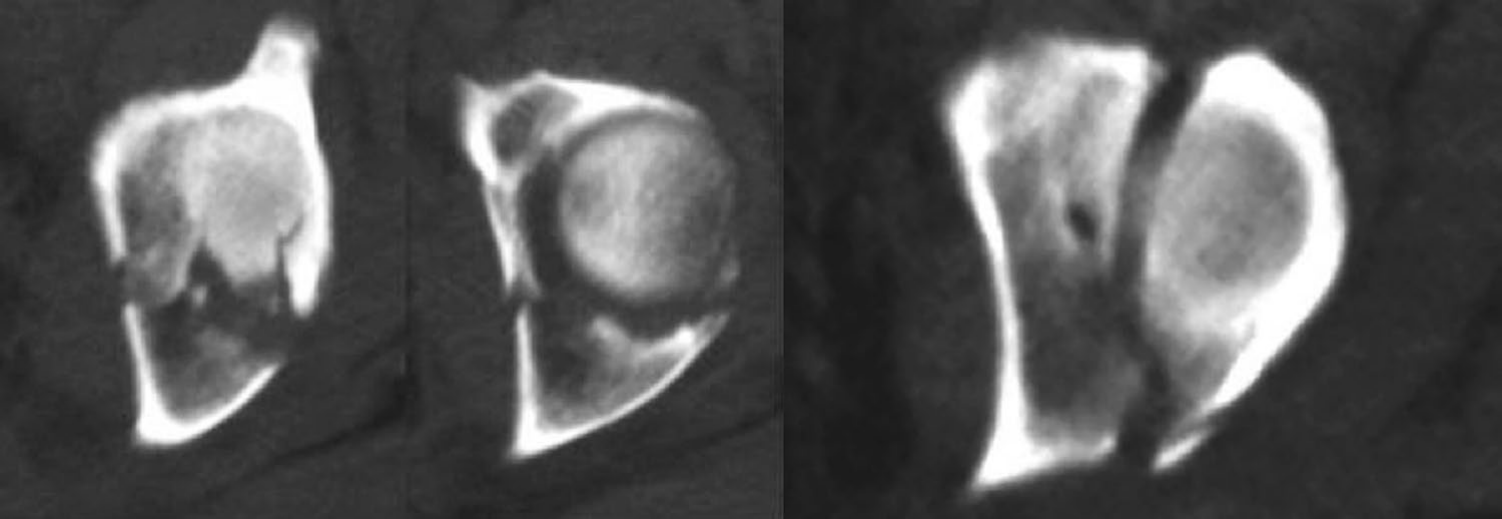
Typical column separation, indicated by a “transverse” fracture line on axial CT (left two CT-views) and a typical “vertical” fracture line indicated by a transverse fracture with the medial fragment being unstable (right CT-view)
